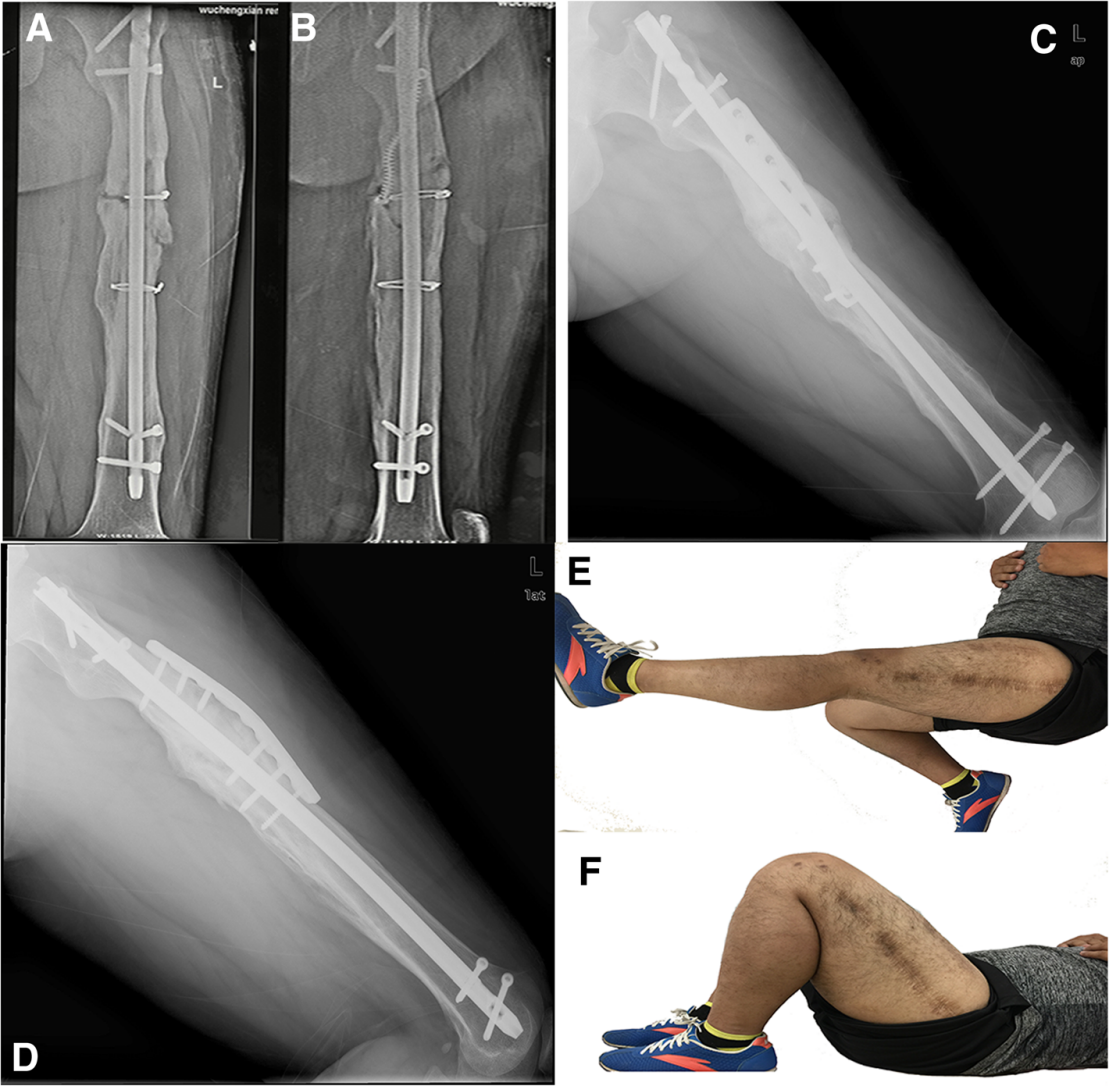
A 26-year-old male with left femoral shaft non-union after nailing. a, b X-ray showing breakage of the distal locking screw with obvious varus deformity and a bone defect (3 cm) preoperatively. c, d X-ray showing fracture healing 6 months after EN/AP. e, f The knee joint function of the affected side was good
